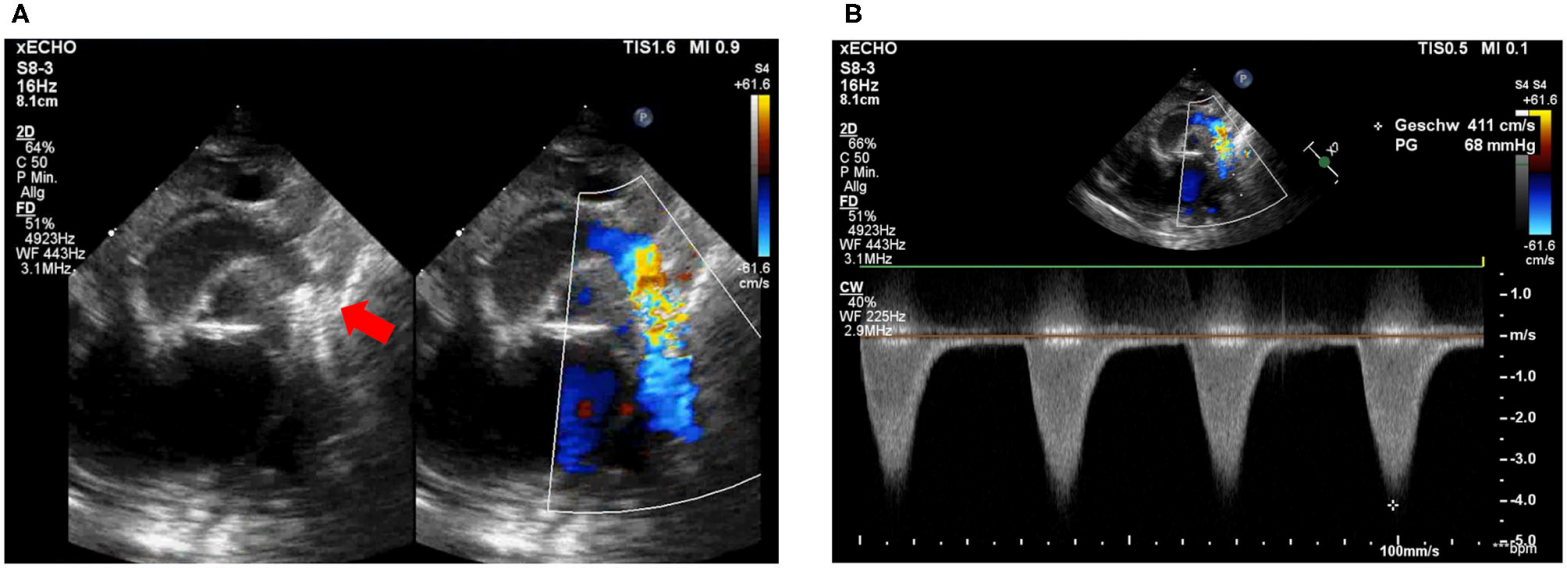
Post-intervention echocardiography. (A) Stent visualization in the descending aorta. Proximal stent struts (red arrow) partially cover the opening of the left subclavian artery (LSA) without obstruction. (B) Flow acceleration over coronary stent in isthmus region with 4.1 m/s.
